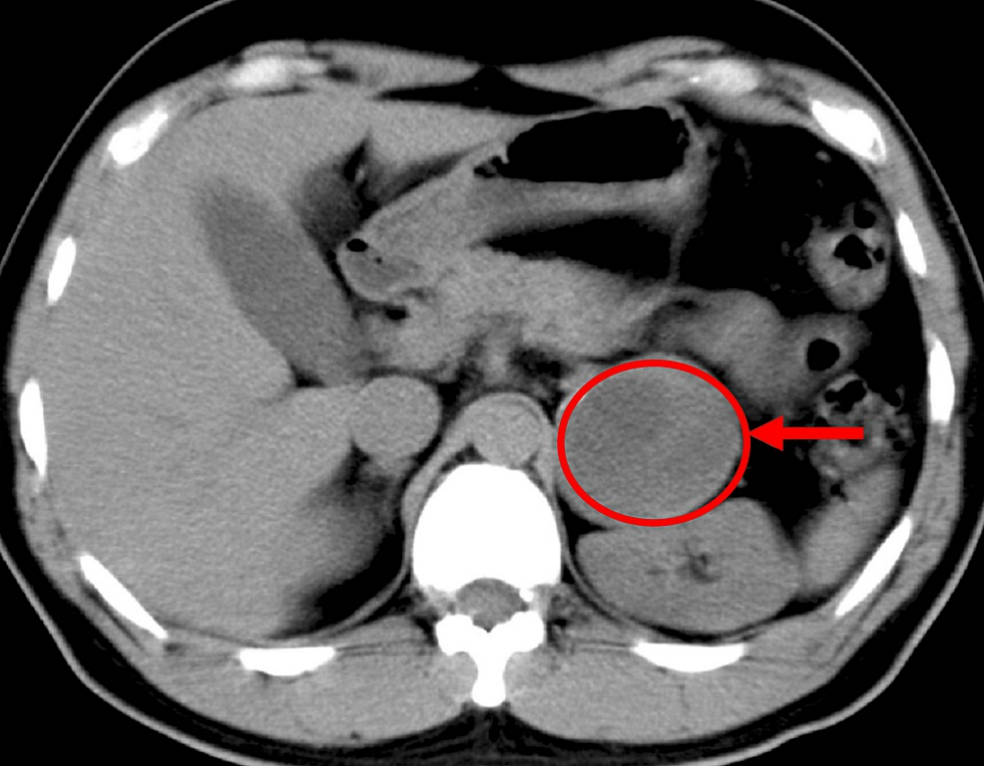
CT of the abdomen An axial view of the CT of the abdomen shows pheochromocytoma located in the left adrenal gland (red circle and arrow).
